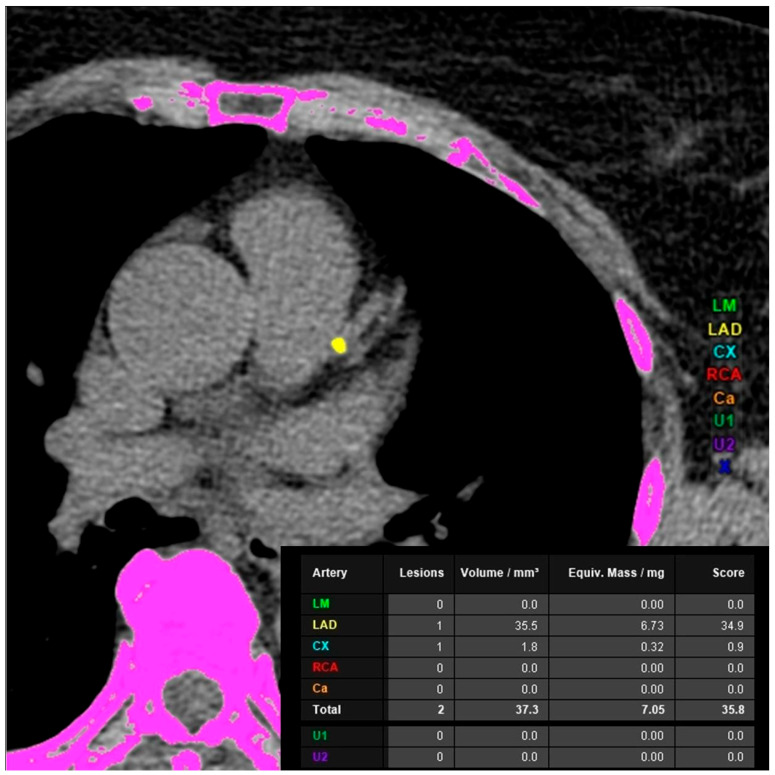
Cardiac computed tomography angiography. Native phase. Coronary artery calcium score assessment—mild risk of significant coronary artery diseases. LM—left main coronary artery, LAD—left anterior descending artery, CX—left circumflex artery, RCA—right coronary artery, Ca—minor branches of the coronary arteries, U—extracoronary calcification.
